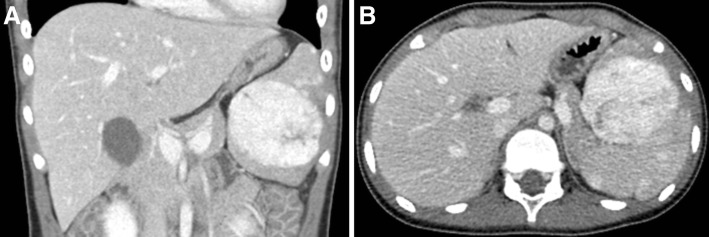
a and b Computed tomography scans shows a giant enhancing mass 61 × 54 × 65 mm in size and several subcentimeter enhancing nodules in the spleen, suggesting possible hemangiomas
